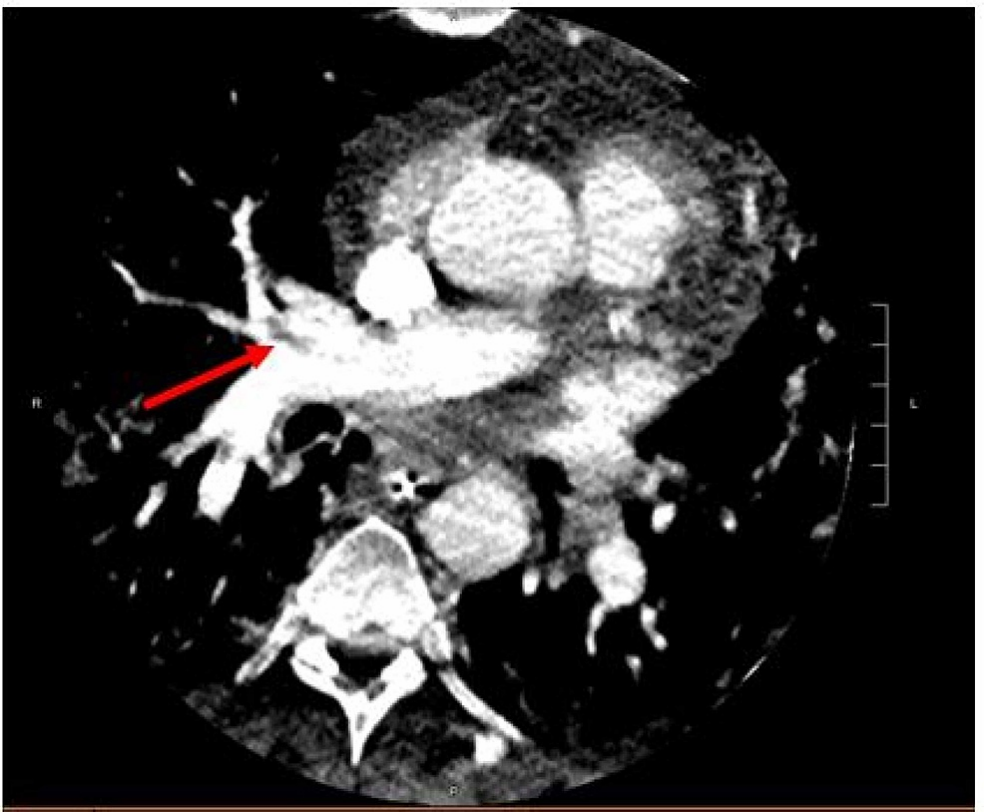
Reconstructed CTA showing upper lobar PE (arrow). CTA, CT angiography; PE, pulmonary embolism
